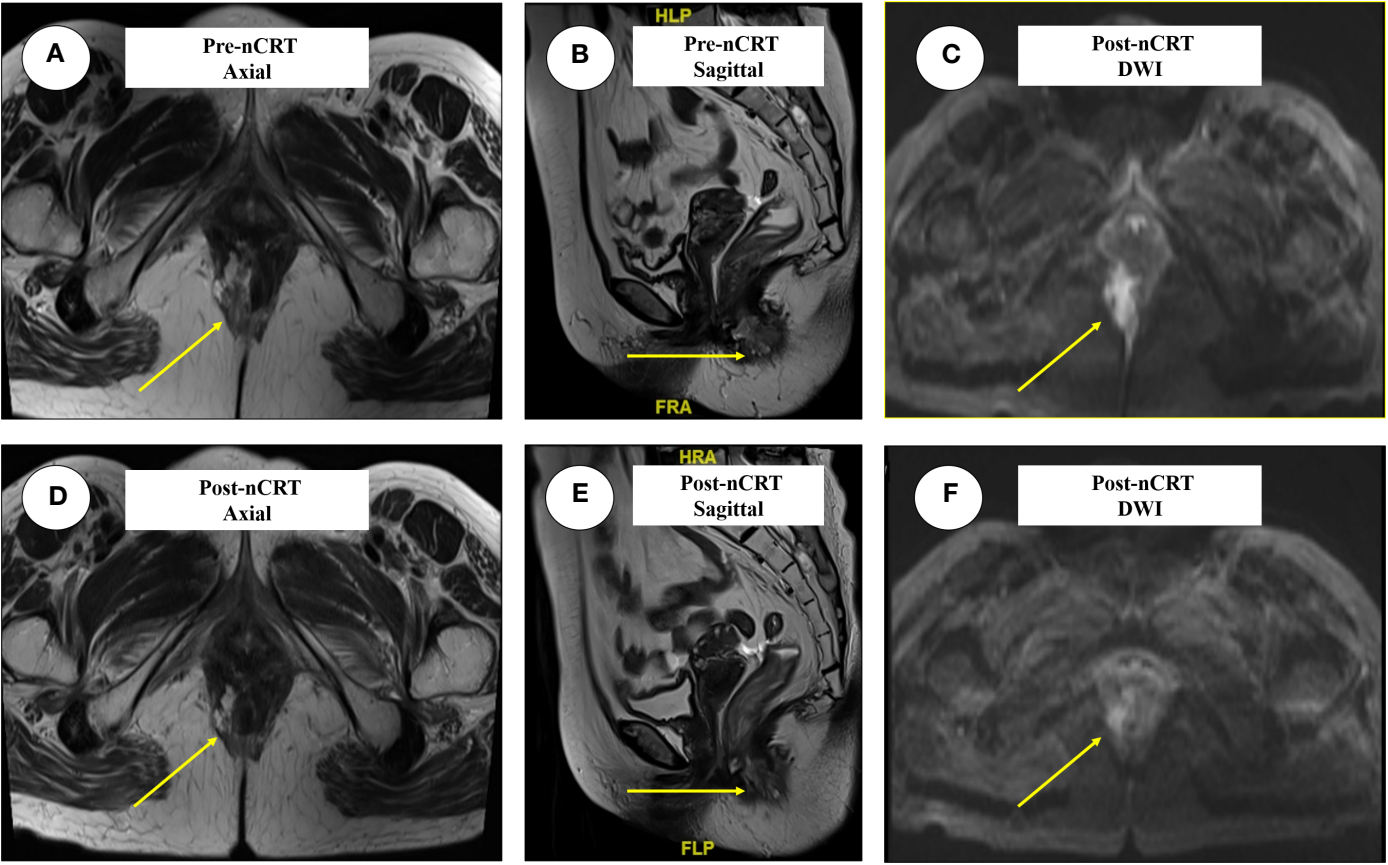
The typical images of anal adenocarcinoma before and after nCRT in three dimensions. The lesions were marked in yellow arrow. (A) The axial images before nCRT, the irregular mass protruding into the intestinal cavity; (B) The sagittal images before nCRT; (C) Lesions presented by DWI before nCRT; (D) The axial images after nCRT; (E, F) represent the sagittal and DWI lesions after nCRT. nCRT, neoadjuvant chemoradiotherapy; DWI, Diffusion weighted imaging; TRG, Tumor regression grade.
